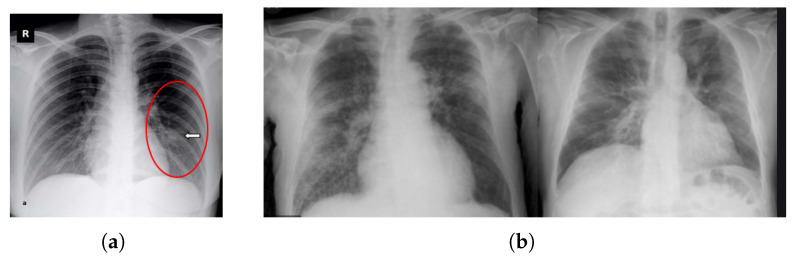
Sample chest X-ray. (a) Ground Glass Opacity found (Opacities of infrahilar airspace were found right side in the red circle of COVID-19 patient) [20]. (b) Consolidation.
